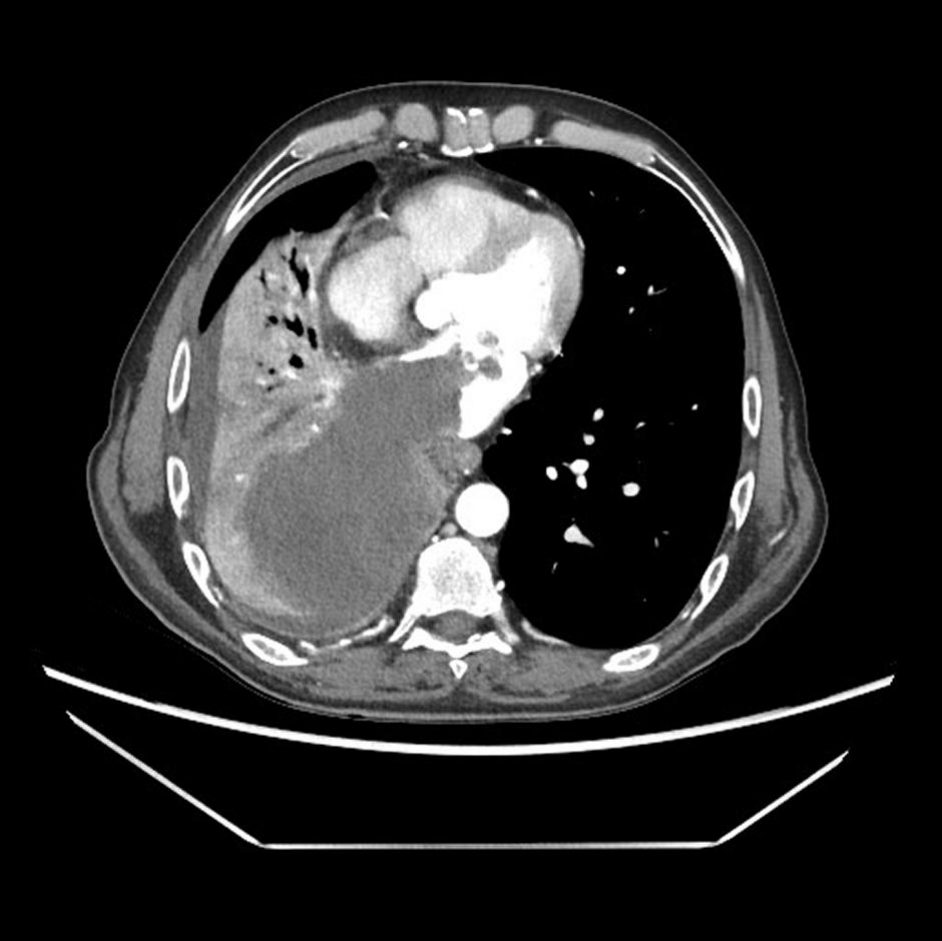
Contrast-enhanced CT scan showing a large necrotic right lower lobe mass invading the left atrium through the right inferior pulmonary vein.
